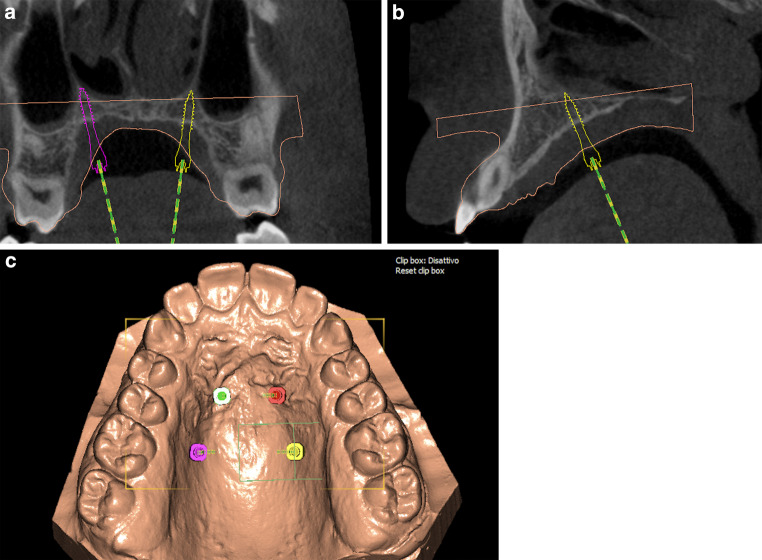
After superimposition of the digitized model and the cone-beam computed tomographic (CBCT) image, the optimal anterior and posterior positions of the mini-implants are virtually planned (Easy Driver V 2.0.2019, Uniontech, Parma, Italy) (case 1) Nach der Überlagerung des digitalisierten Modells und der DVT-Aufnahme wird die optimale anteriore und posteriore Position der Mini-Implantate virtuell geplant (Easy Driver V 2.0.2019, Uniontech, Parma, Italien) (Fall 1)
